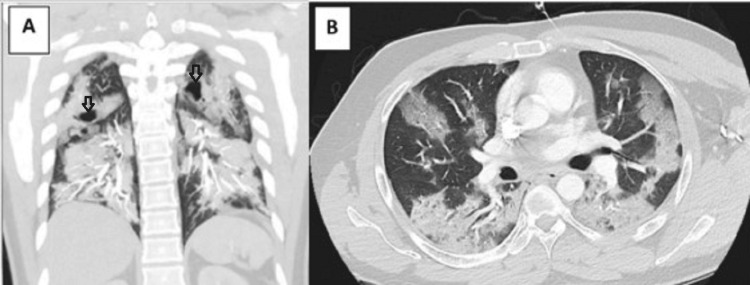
Coronal and axial CT imaging showing extensive consolidation and ground-glass opacities bilaterally with pneumatoceles (arrows) A: Coronal CT demonstrating extensive consolidation and bilateral pneumatoceles (arrows) B: Axial CT showing extensive multi-lobar consolidation with ground-glass opacities CT: computed tomography
